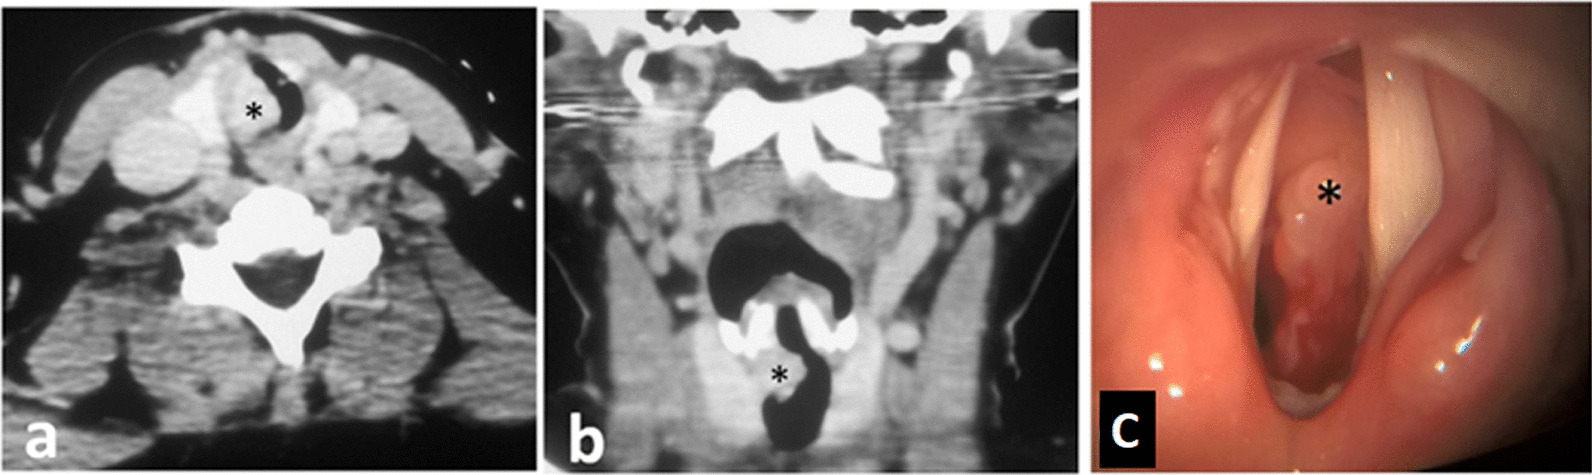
a-axial CT showing the soft tissue mass (*) occluding the tracheal lumen. b coronal CT showing the mass (*) extending from the lower border of the cricoid cartilage till the 3rd tracheal ring. c direct laryngoscopy showing soft tissue mass (*) below the vocal cords obstructing more than 80% of the airway lumen
